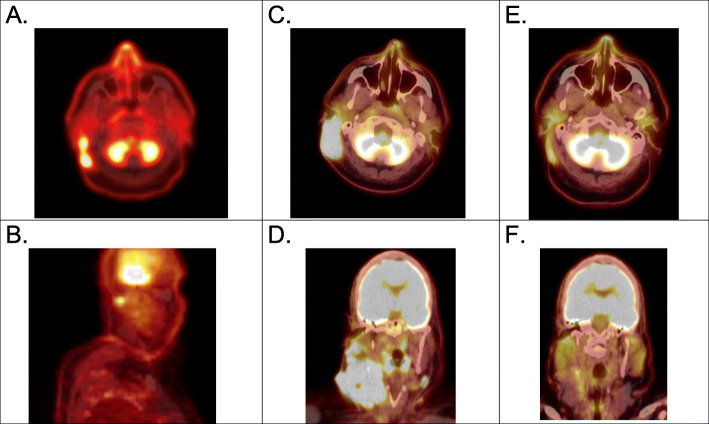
PET/CT scans showing the clinical response of patient 8: Initial pretreatment scan showing melanoma metastatic to (A) retro-auricular scalp soft tissues and (B) a small ipsilateral neck node. In (C and D) similar cuts show dramatic worsening of postauricular disease and bulky neck lymph nodes after 4 cycles of ipilimumab plus nivolumab therapy. Clinical response to combination CKI + TT is shown in scalp lesion E), as well as the neck adenopathy (F) at 40 months from start of CKI. Due to residual low-level fluorodeoxyglucose uptake at both neck and post-auricular sites, both were biopsied, and a pathologic complete response was documented. The patient remains stable at over 9 months after discontinuation of all treatment
